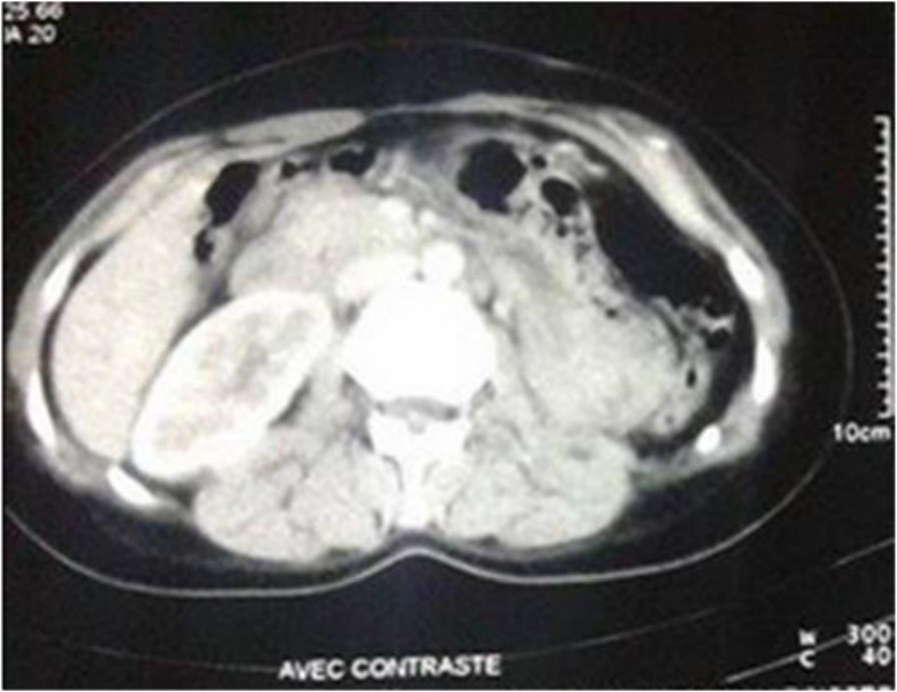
Axial computed tomography performed 5 months after radical nephrectomy showing a heterogeneously enhanced mass of 6 cm in the nephrectomy bed
